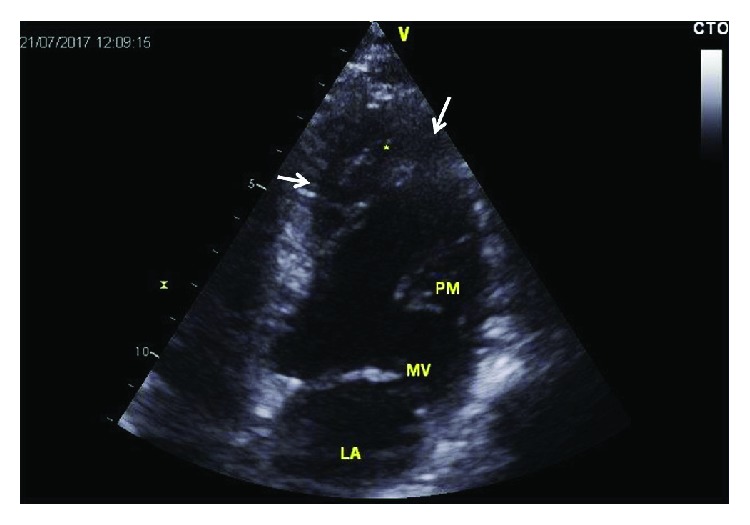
Echocardiographic apical five chamber view from 2017 showing hypertrabeculation/noncompaction in the apex (arrows). LA: left atrium; MV: mitral valve; PM: anterolateral papillary muscle.
